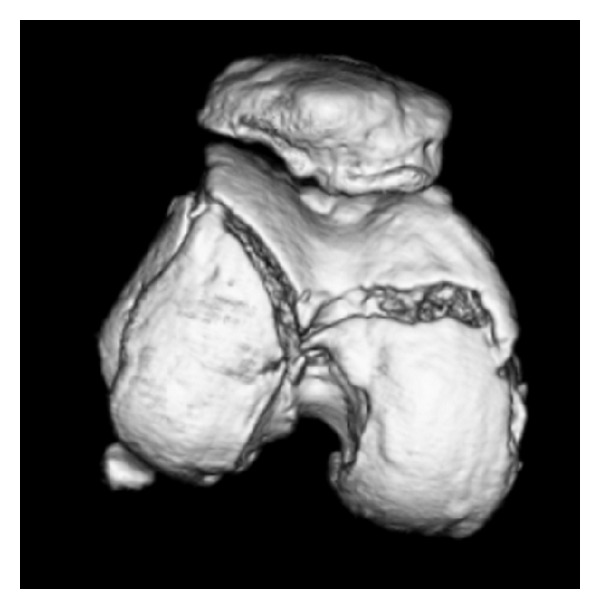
A three-dimensional computed tomographic image of the femoral articular cartilage of the right knee, showing the bicondylar Hoffa fracture.
